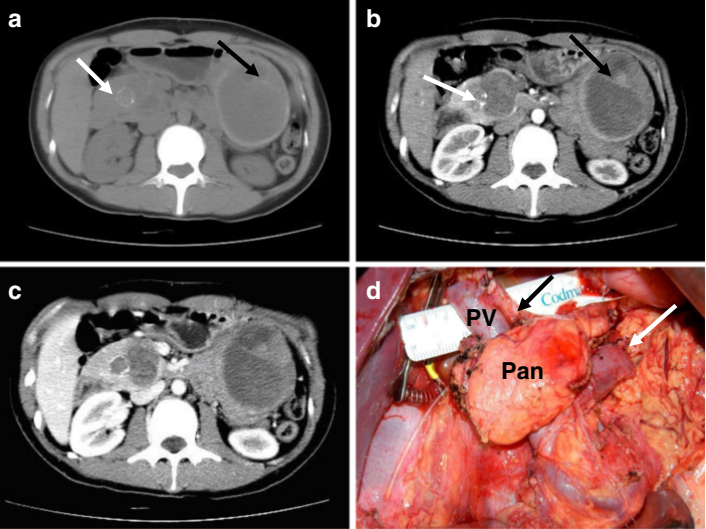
CT scans and intraoperative imaging of patient one. a) Plain CT revealed two well-defined heterogeneous lesions: calcification was shown in the pancreatic head tumor (white arrow) and a septum was shown in the tail tumor (black arrow). b) Enhanced CT showed peripheral enhancement and complex cystic components with areas of necrosis; calcification (white arrow) and septum (black arrow) were clearer (arrow). c) Enhanced CT scan in portal phase. d) After a simultaneous atypical PD and atypical LP, about 6 cm of the pancreatic body was preserved (Pan), proper hepatic artery (black arrow) and the stapled stump of the splenic vein (white arrow) can be seen. D, duodenum; G, gallbladder; Pan, Pancreas; T, tumor.
